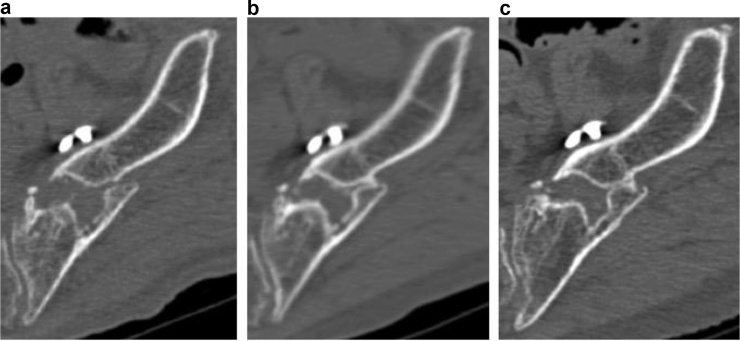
Post-operative computed tomography axial views. Computed tomography images showing the process of union of the fracture site at (a) 5, (b) 12 and (c) 19 months after surgery reveal that union occurred between 12 and 19 months post-operatively.
